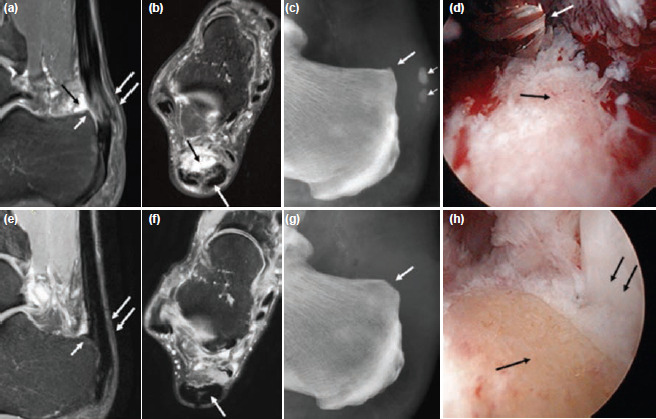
(a) Sagittal and (b) axial proton density fat suppressed (PDFS) MR images of the right ankle showing Haglund's triad with Haglund deformity (short white arrow), retrocalcaneal bursitis (black arrow) and insertional Achilles tendinopathy (double white arrows). Hyperintense signal and interstitial split tears were noted at the distal Achilles tendon (long white arrows). (c) Lateral ankle radiograph shows Haglund deformity (white arrow) and calcifications in the retro-Achilles bursa (small arrows). (d) Posterior ankle arthroscopic view from the medial portal showing the Haglund deformity (black arrow) and a 4-mm arthroscopic burr (white arrow) from the lateral portal. (e, f) Similar MR images as in (a, b) six years following surgery with burring of previous Haglund deformity (short white arrow), showing repair and regeneration of the Achilles tendon with reversal of the pathology to signal normality (long white arrows). (g) Lateral ankle radiograph following arthroscopic burring of the Haglund deformity (white arrow) and removal of the calcifications in the retro-Achilles bursa. (h) Arthroscopic view similar to (d) following arthroscopic burring of the Haglund deformity (black arrow) revealing the previously impinged Achilles tendon (double black arrows).
